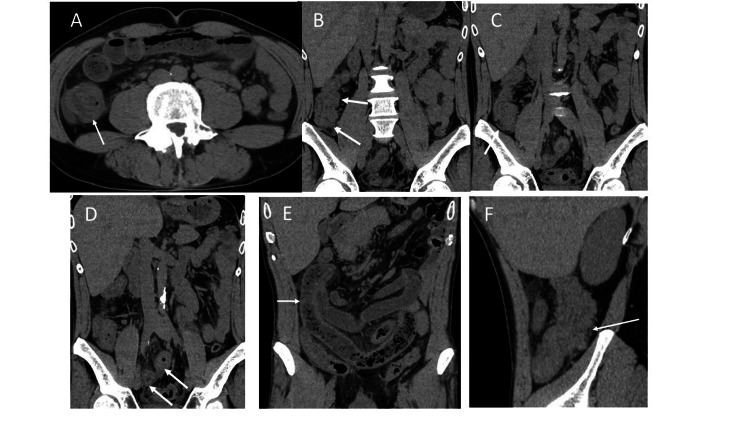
An abdominal computed tomography images of this case The abdominal CT images showed the intussusception of the ileum, and a dilated oral ileum was observed. (A, B) Arrows represent the intussusception image; (C) arrows represent the dilated ileum oral of the intussusception segment; (D) arrows represent the thickening ileal walls; (E) arrows represent the feces in the oral ileum of the intussusception segment; (F) arrows represent the incarcerated ileum in the ascending colon.
